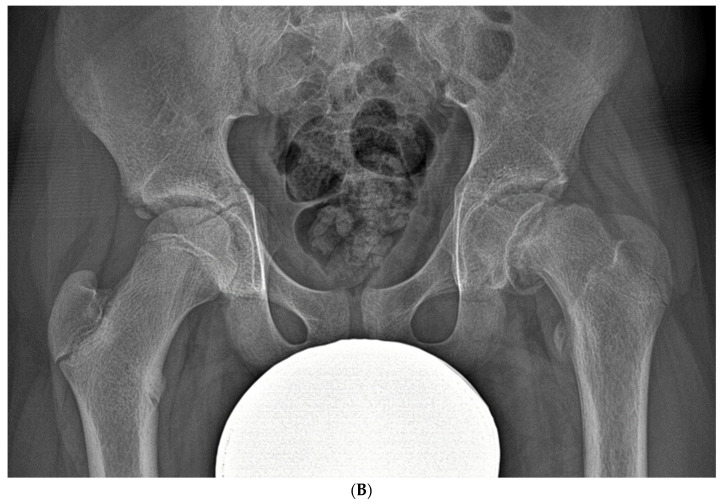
(A) A case of exfoliation of the femoral head in a 13-year-old boy. Images are in the plane of the long axis of the femoral neck. Image of healthy right hip joint (right); exfoliation of the left femoral head (left). Arrows—level of growth plate of the femoral head; arrowheads—proximal femoral epiphysis. Accompanying exudate (dotted line) is visible in the left hip joint. Linear probe 3–19 MHz. Figure 14. (B) X-ray image of the same patient. Visible exfoliation of the left femoral head.
